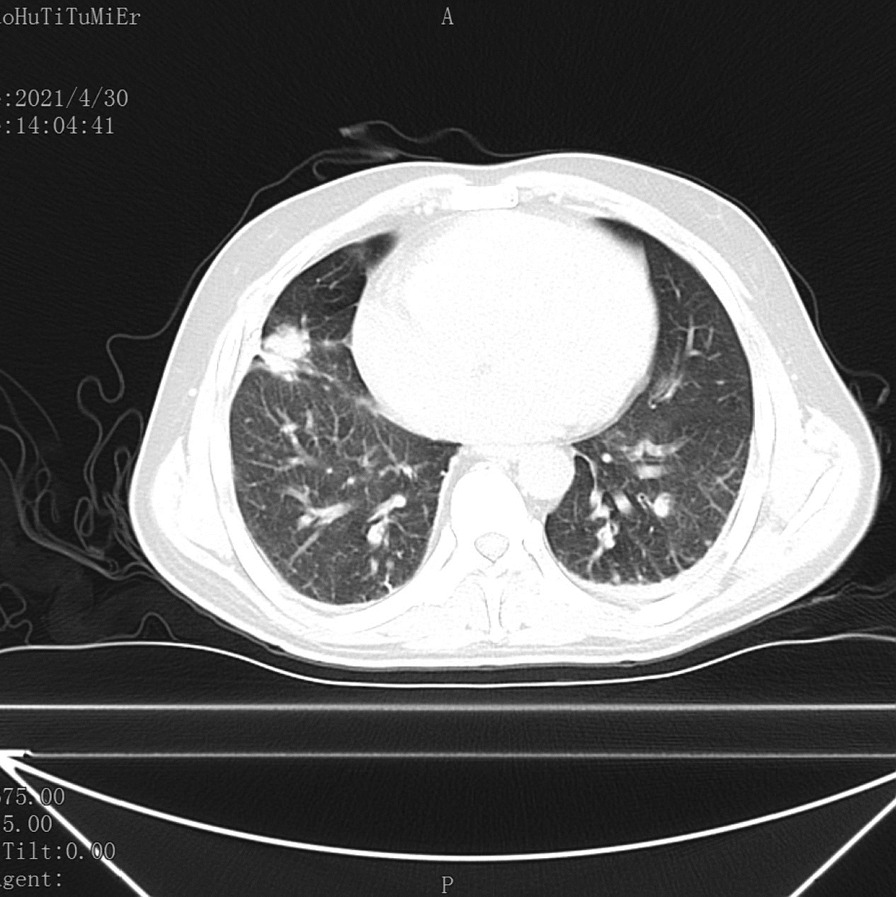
Preoperative lung Computed tomography (CT) showed a round mass in the middle lobe of the right lung, and the primary lesion was considered to be lung cancer
